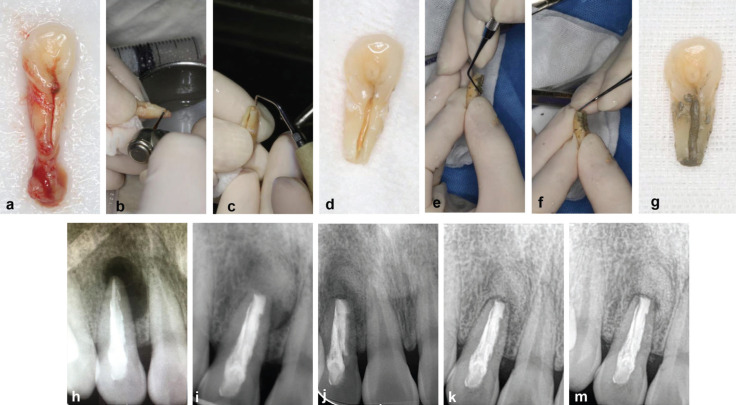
(a) The extracted maxillary right lateral incisor with apical granuloma was placed in a sterile gauze soaked with saline solution. (b) the manipulation of apexectomy. (c) the manipulation of retrograde preparation. (d) The palate-gingival groove was prepared with a fissure bur, and the apical 3 mm of the root apex was resected and retrogradely prepared. (e) the palate-gingival groove was filled with MTA. (f) the root apex was filled with MTA. (g) the tooth presented that both the palate-gingival groove and the root apex were filled with MTA. (h) Preoperative radiograph. (i) Radiograph taken after treatment (the apical region was filled with Bio-oss). (j) Radiograph taken at 2-month follow-up (k) Radiograph taken at 6-month follow-up (similar density with the surrounding bone tissue in the apical area and the obvious periodontal healing) (m)Radiograph taken at 12-month follow-up (similar density with the surrounding bone tissue in the apical area and the obvious periodontal healing).
